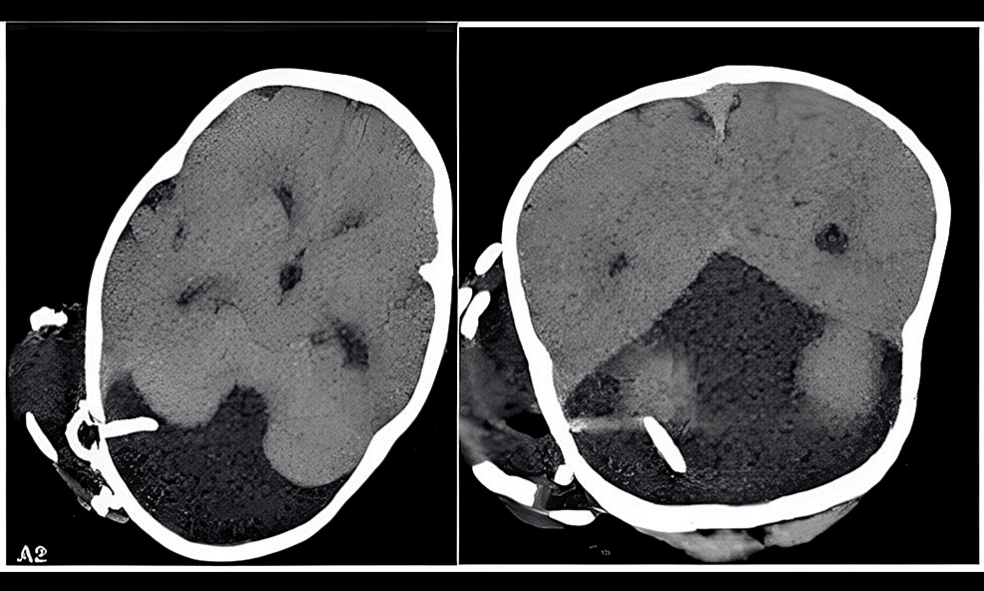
Brain CT scan finding; There is a right occipital approach.
